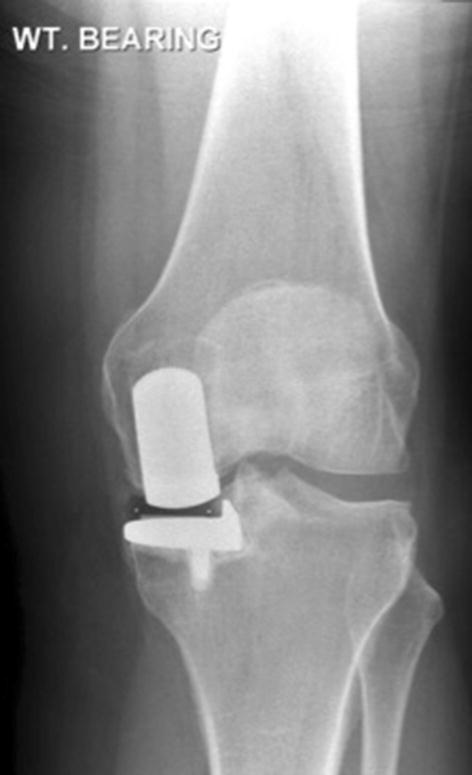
A malaligned AP film with a possible radiolucency. The only way to determine if there is a radiolucency and if it is pathological or physiological is with an aligned radiograph
